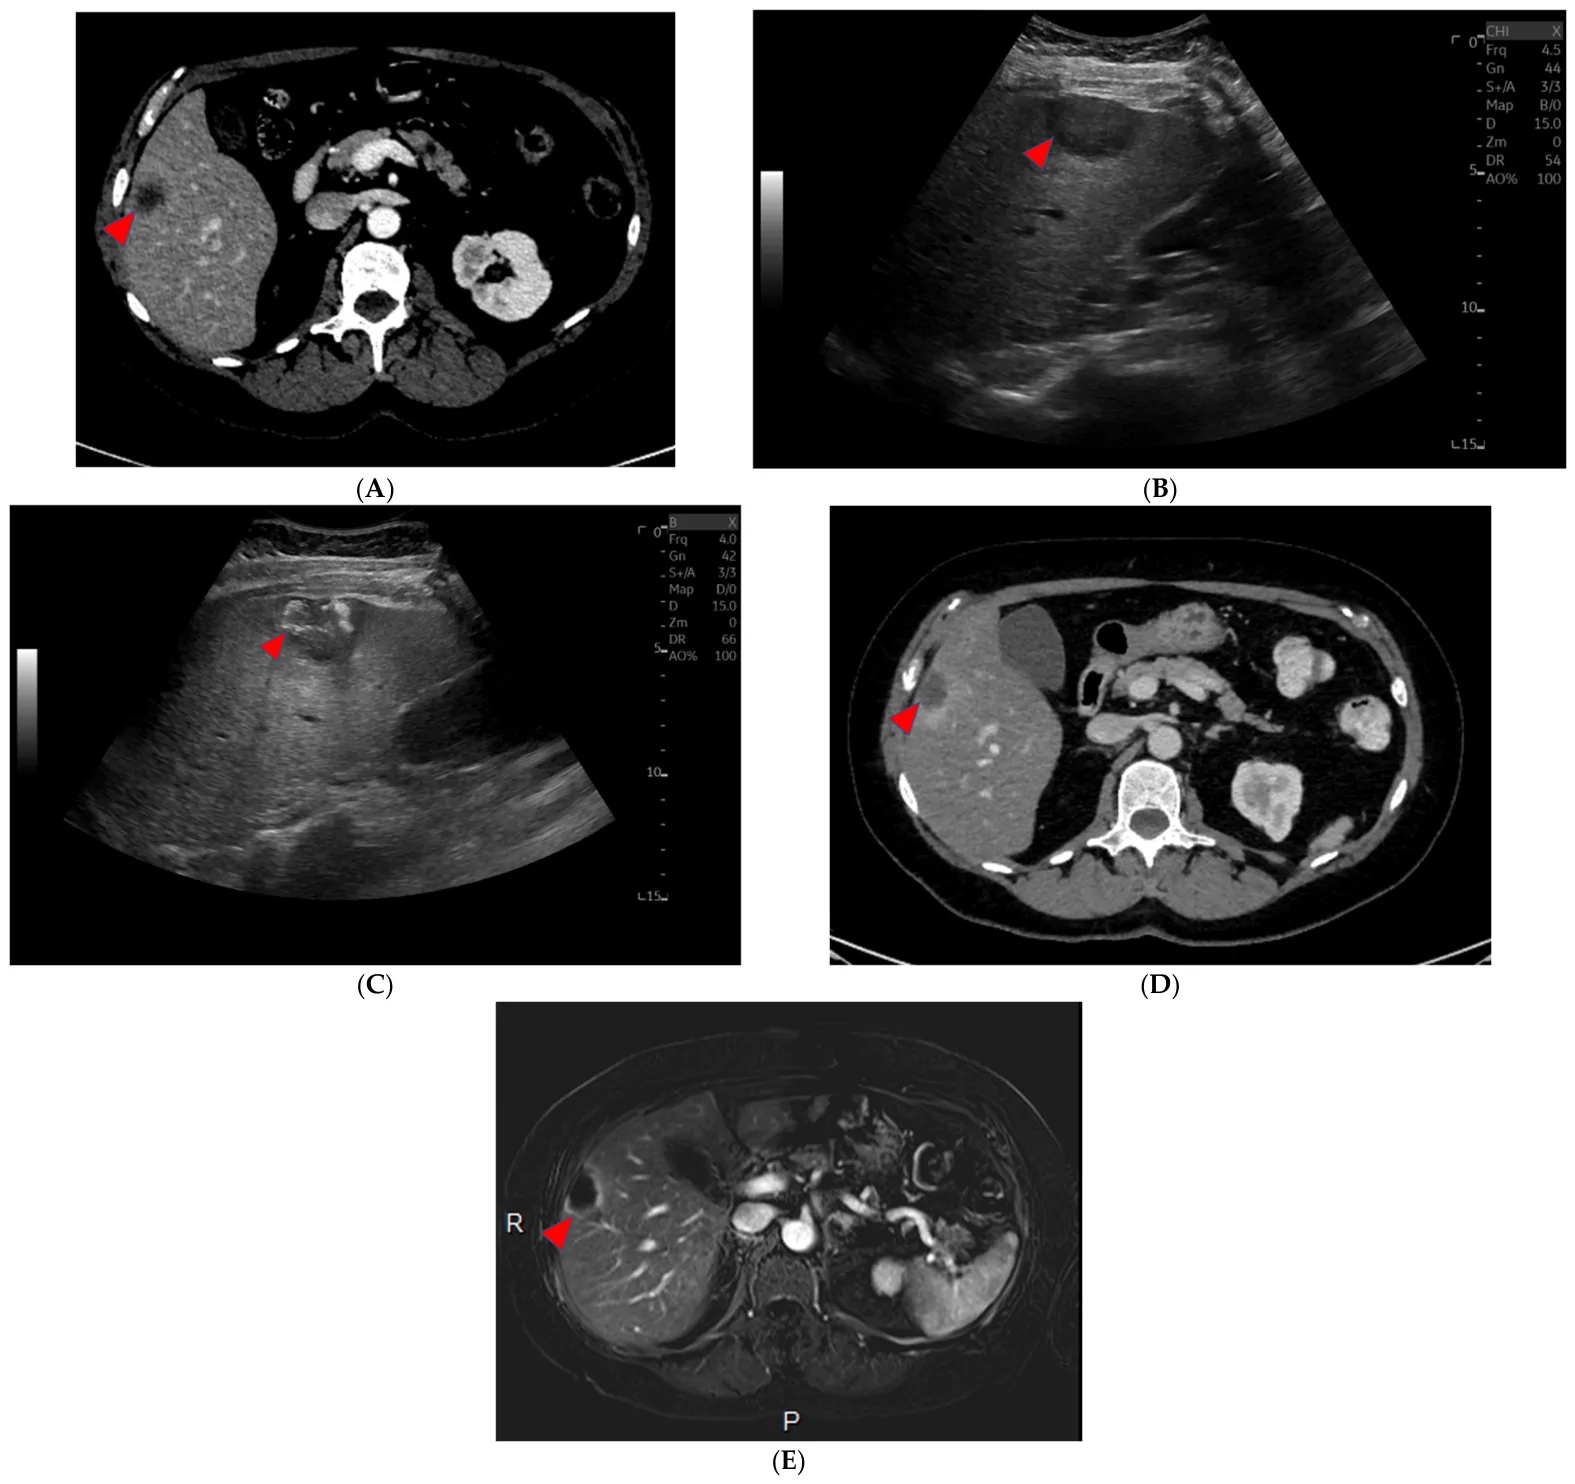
(A–E): Institutional review board approval was not required for this de-identified patient case. A 67-year-old female with history of oligometastatic breast cancer with a solitary liver metastasis (2.5 cm) that was enlarging despite treatment with systemic ribociclib was referred for histotripsy with a plan to initiate new systemic therapy post treatment. Per our standard work-up, the patient was evaluated for the tumor size, number and location and the potential for alternative therapy options. A screening ultrasound was performed in clinic to ensure the lesion could be treated with histotripsy. (A) Contrast-enhanced (CE) CT demonstrates a hypoenhancing solitary mass in hepatic segment 5 (arrowhead). (B) Planning preprocedural grayscale ultrasound image demonstrates a capsular-based hypoechoic mass (arrowhead) correlating to the tumor seen on CT imaging. (C) Immediate post-histotripsy grayscale ultrasound imaging demonstrates expected acoustic shadowing and echogenic foci of gas in the treatment volume (arrowhead). (D) One-month CE CT scan image demonstrates the rim-enhancing mass without complicating features (arrowhead). (E) Subtracted CE T1 weighted MRI demonstrates thin rim enhancing treatment zone (arrowhead), 2 months post-therapy. For image orientation, R = right side of patient and P = posterior/back of patient.
